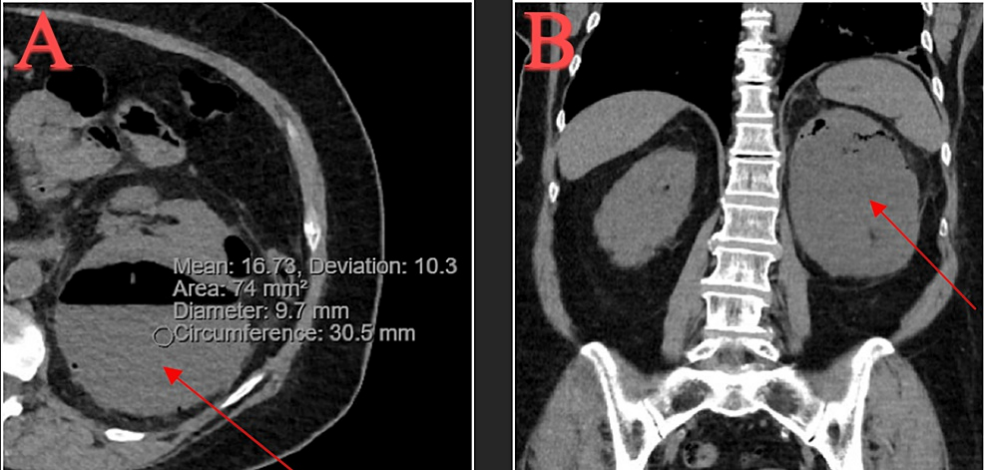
CT abdomen and pelvis w/o contrast of left kidney subcapsular hematoma: (A) transverse view and (B) coronal view. CT, computerized tomography
